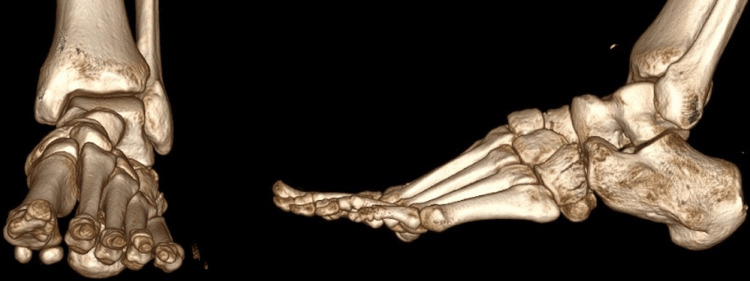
3D foot CT showing dorsal dislocation of the intermediate cuneiform
